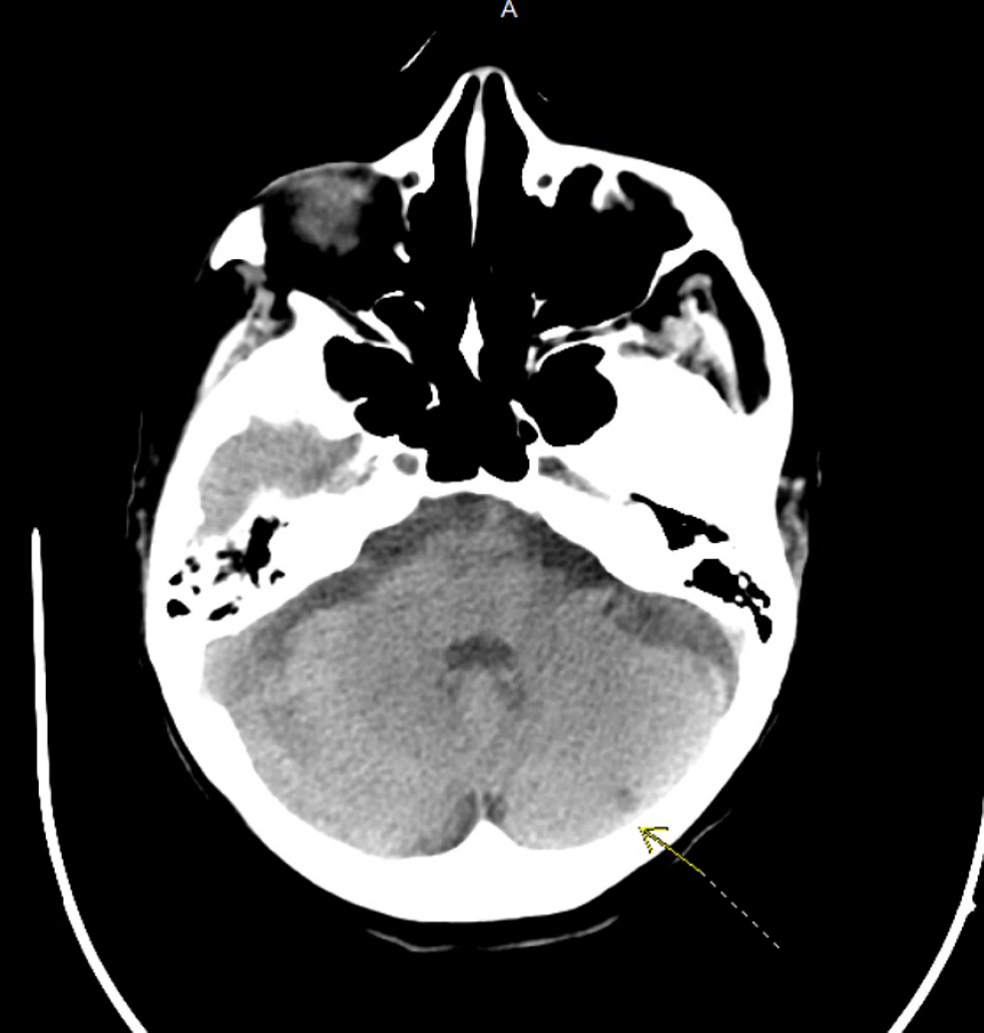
CT head without contrast showing a thin, predominantly low-density sub-acute to chronic fluid collection (yellow arrow) along the inferior left frontal lobe with mild mass effect on adjacent parenchymal structures
